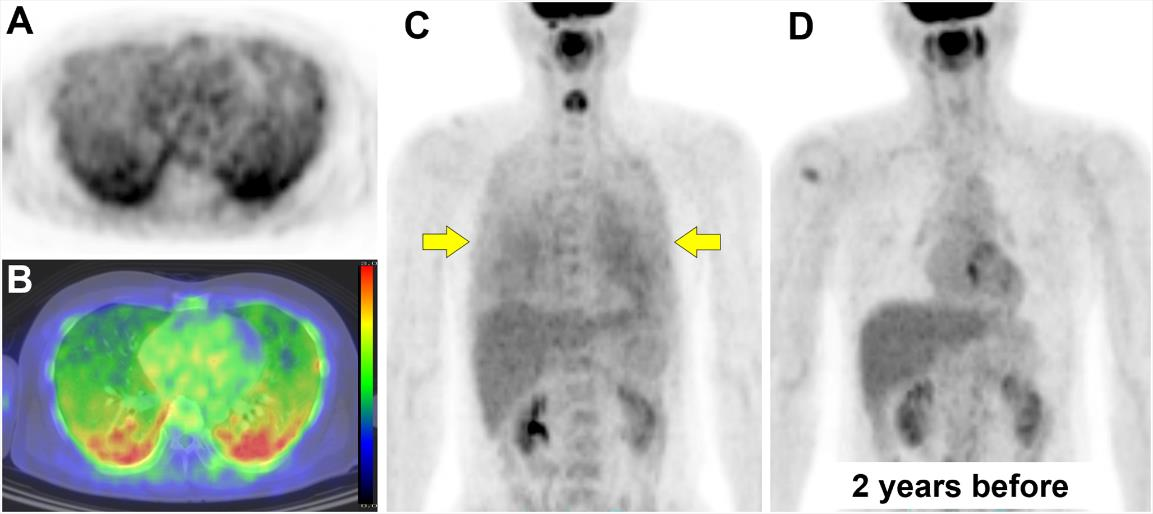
On axial images of PET (A) and PET/CT fusion (B), dorsal-dominant diffuse pulmonary uptake was confirmed. Maximum intensity projection image showed diffuse uptake in the bilateral lungs (C, arrows), which was not confirmed 2 years before (D)
